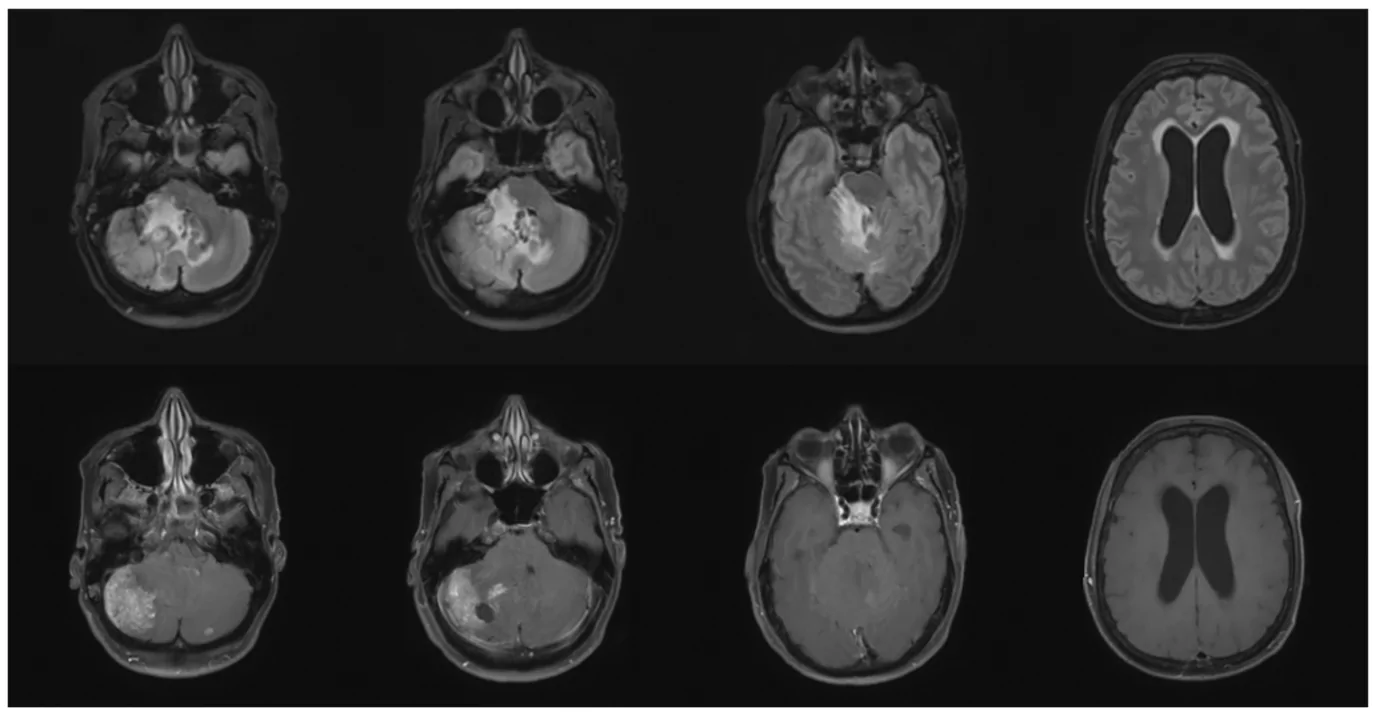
Brain MRI at diagnosis of neurological symptoms: Upper row: axial T2-FLAIR sequences. Lower row: axial T1-weighted sequences after gadolinium injection.
